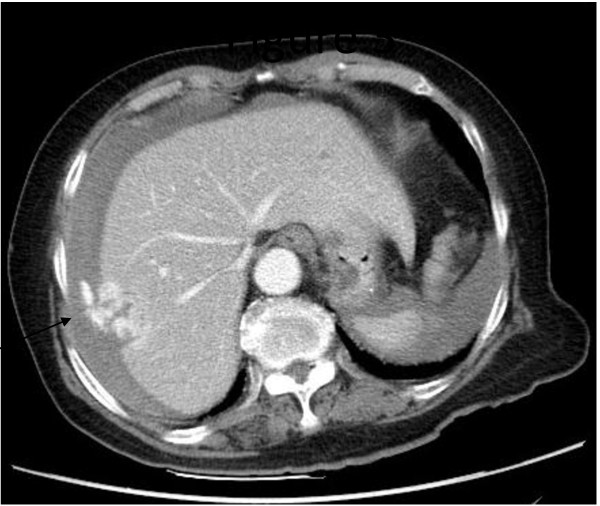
Enhanced CT scan showed a ruptured irregular liver mass. Enhanced CT scan showed a ruptured irregular liver mass (balck arrow) with prominent enhancement at the liver surface inducing contrast leakage into the right subphrenic space.
